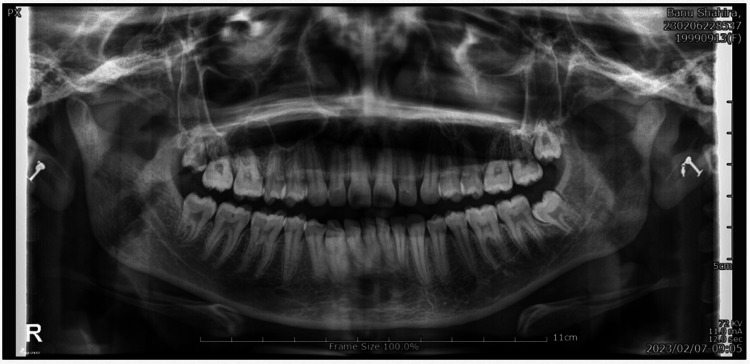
Digital OPG used for assessment OPG: Orthopantomograph
